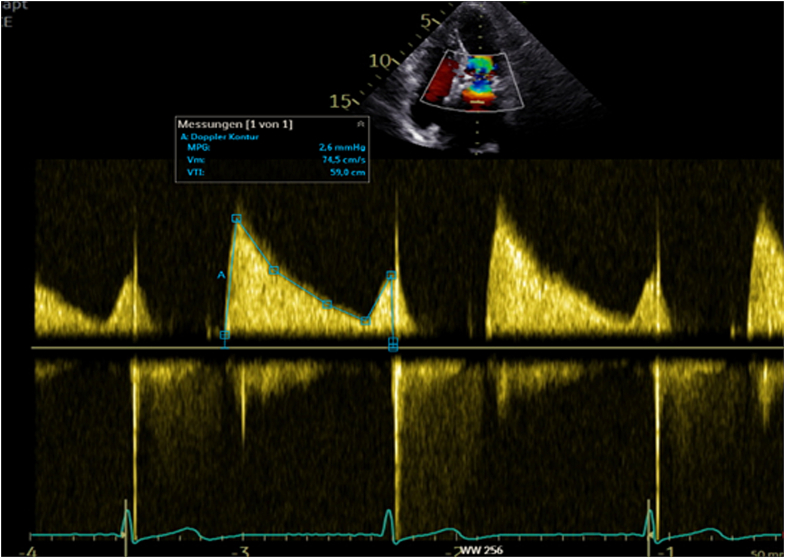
Transthoracic Echocardiography After Intervention Transthoracic echocardiography of the mitral valve after valve-in-valve procedure; continuous-wave Doppler across the mitral valve shows a mean transmitral gradient of 2.6 mm Hg.
